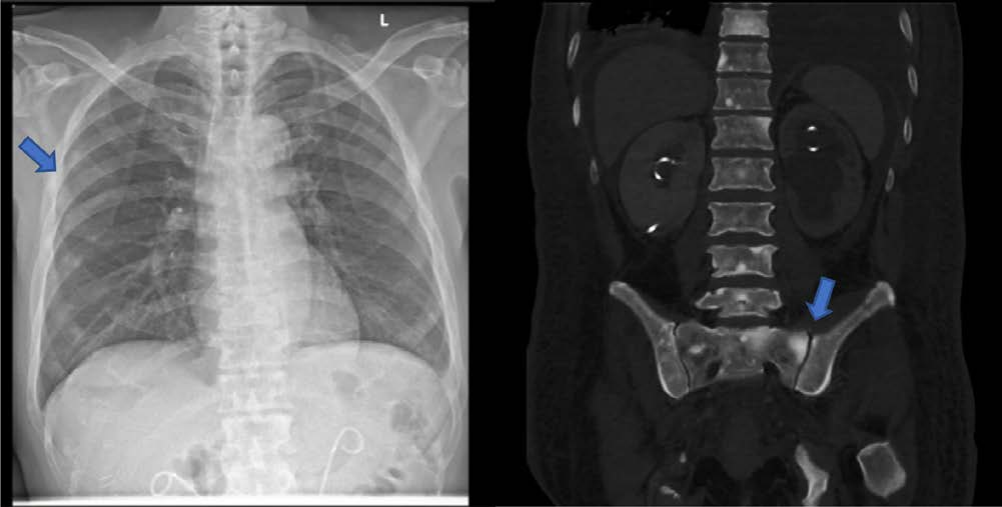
Chest radiography (CXR) and computed tomography (CT) scan showing extensive osteoblastic bone metastases (blue arrows).
